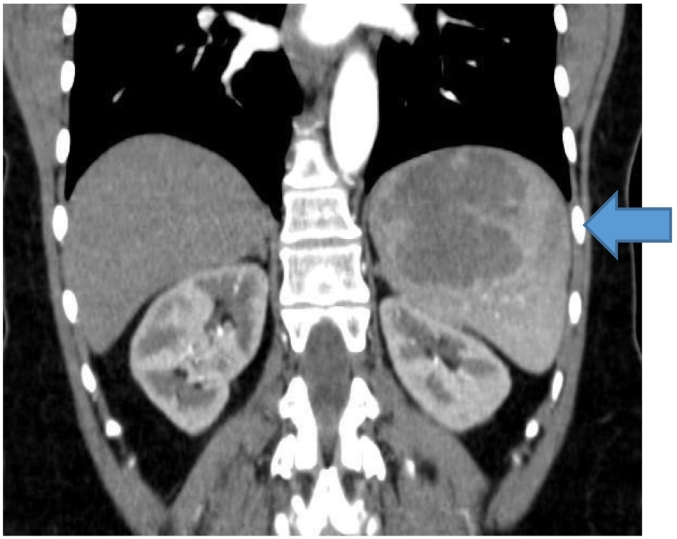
Coronal CT abdomen scan with the arrow demonstrating the mass in the superior pole of the spleen.
